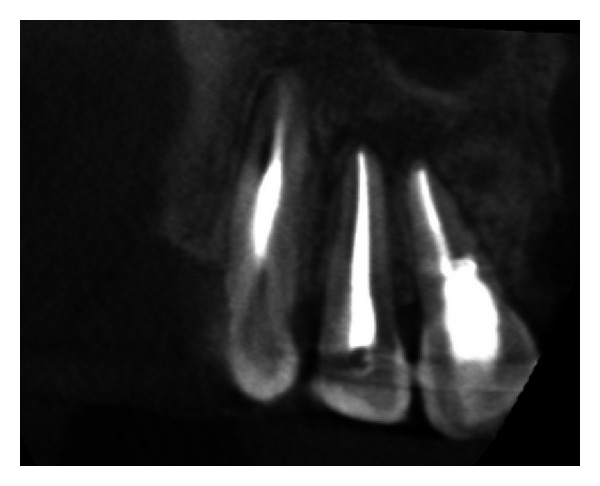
1-year followup CBCT image in coronal plane.
